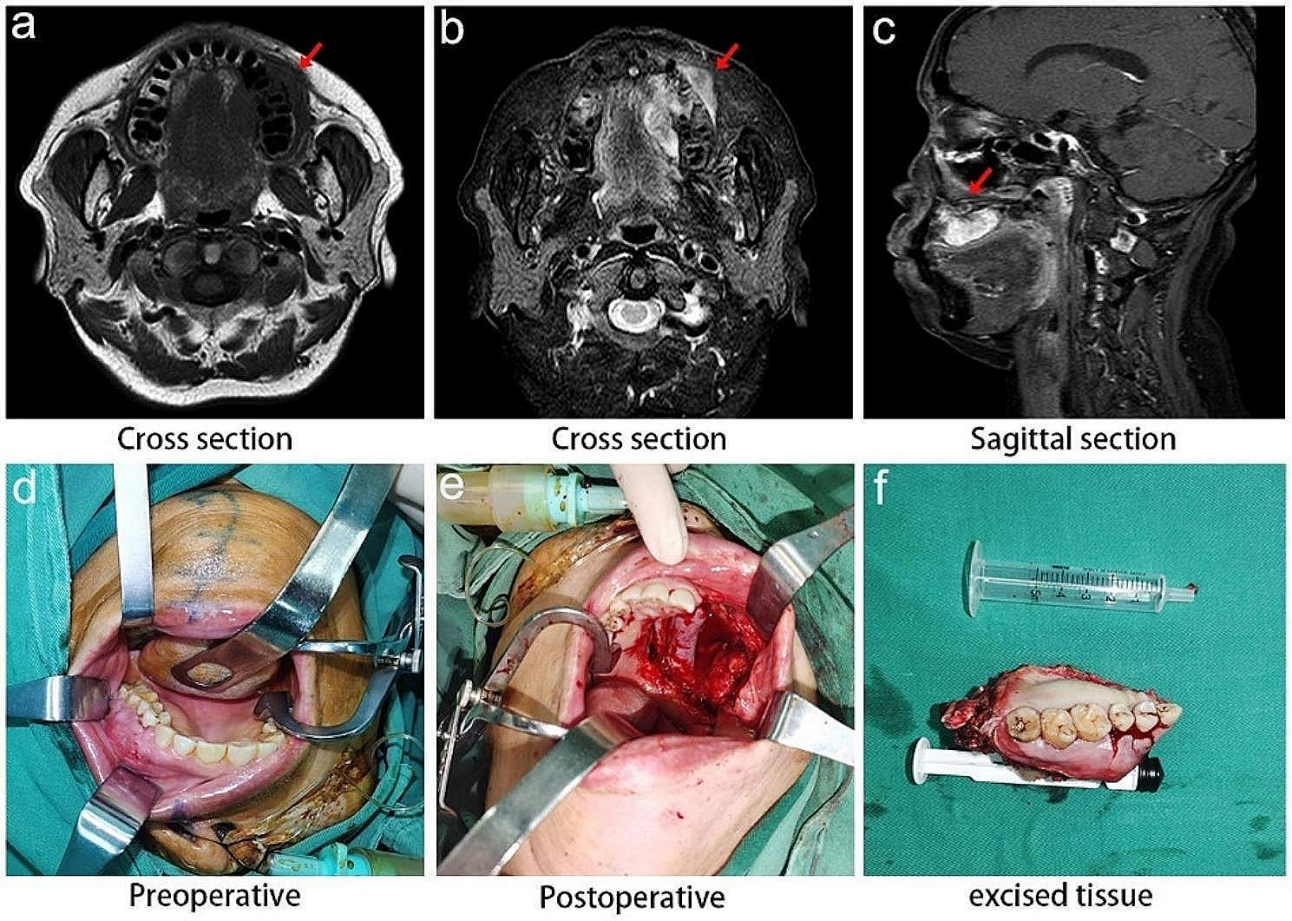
MRI imaging of the mass in the left maxilla and macroscopic findings with intraoperative observations of the tumor. (a) and (b) Cross-sectional MRI of the maxillary mass. (c) Sagittal section imaging of the maxillary mass under MRI. (d) Intraoral image of the tumor before surgery. (e) Intraoral image of the tumor after surgery. (f) The surgical maxillary fragment
